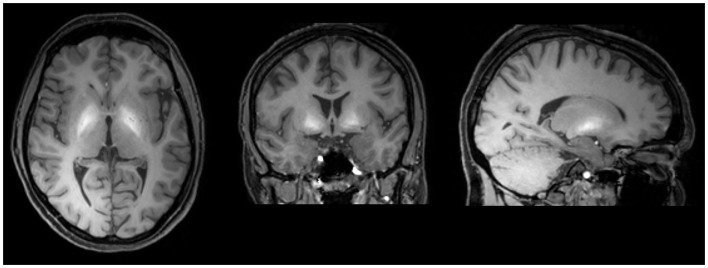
T1 weighted MRI brain scan of a methcathinone abuser, showing high intensity areas of manganese deposits in the basal ganglia. The deposits disappear after cessation of substance abuse while the extrapyramidal symptoms are irreversible.
